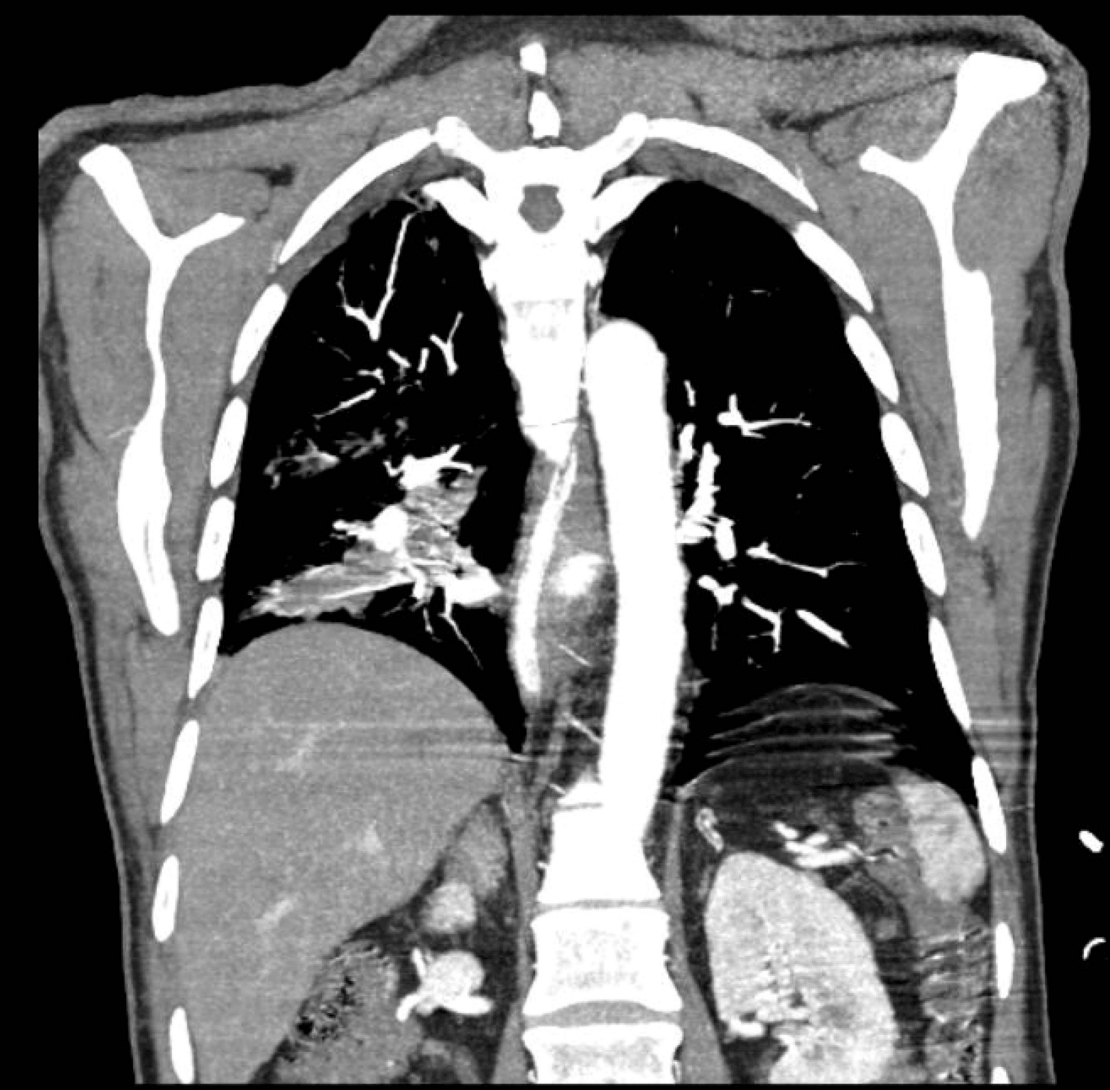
CT showing a lower right lobe 32 mm lesion
